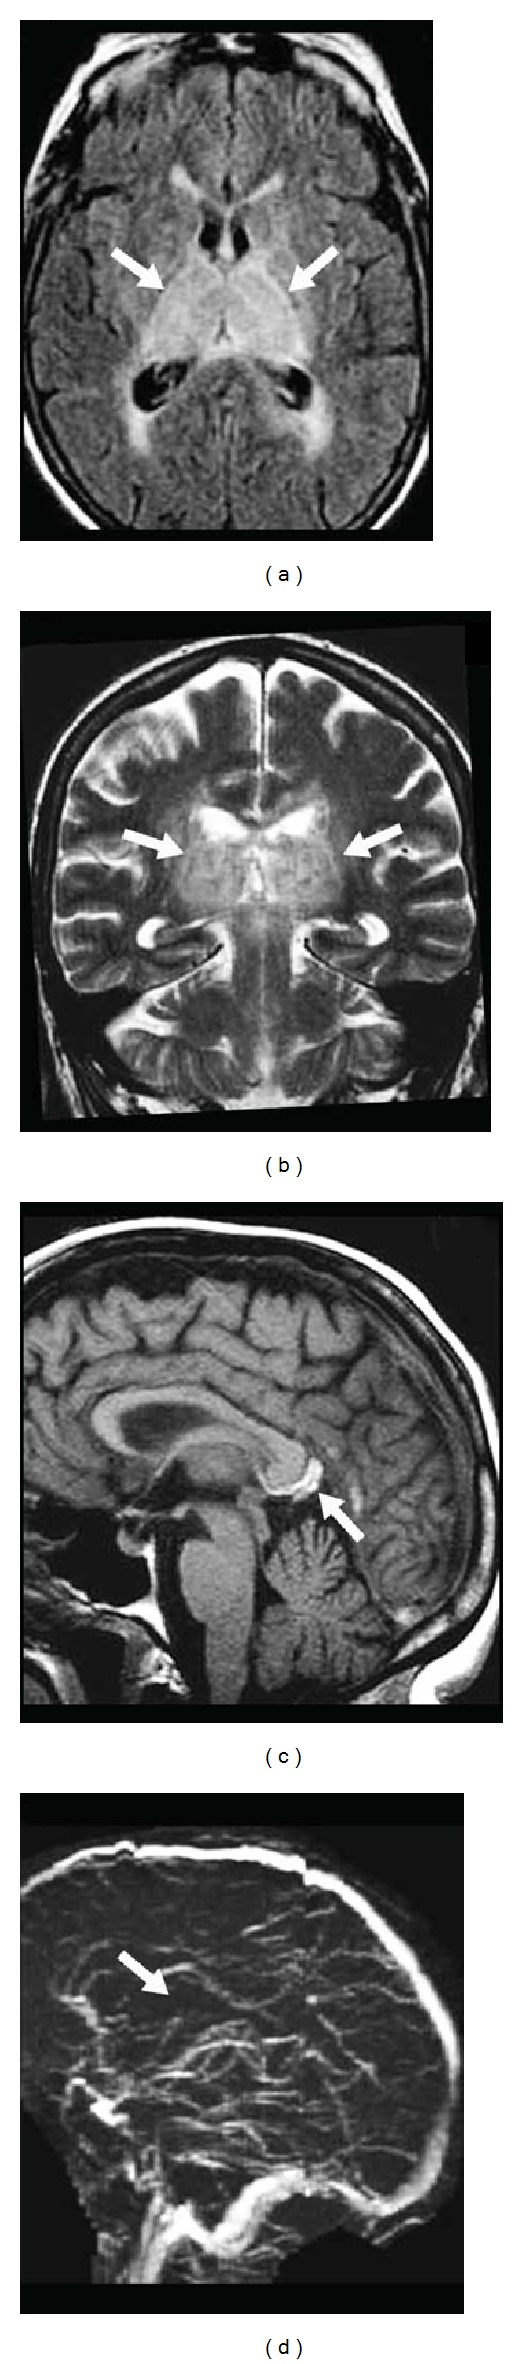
Bilateral thalamic vasogenic edema seen as hyperintensity on both axial FLAIR (a) and coronal T2-weighted (b) imaging due to venous thrombosis of the deep cerebral venous system. The venous thrombosis of the vein of Galen is seen as hyperintensity on sagittal unenhanced T1-weighted imaging (c), and lack of flow in the deep cerebral venous system is seen on MR venography (d).
